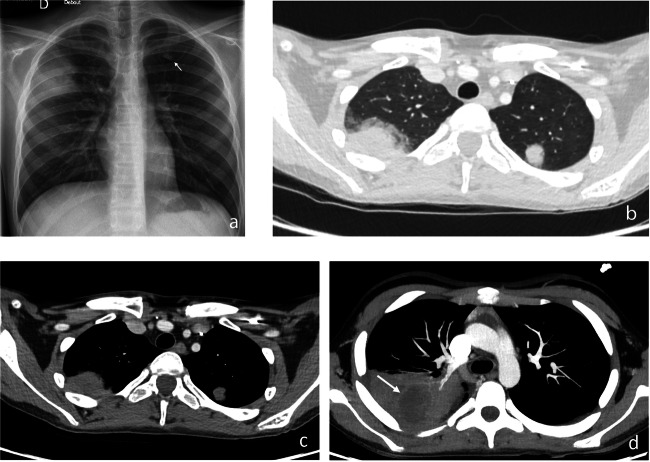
Proven invasive pulmonary aspergillosis in a 16-year-old boy with acute myeloblastic leukemia (AML) under chemotherapy. Chest X-ray (a) performed because of increasing thoracic pain after minor trauma evidences an extensive lung infiltrate with ill borders in the right upper lobe and a nodule below the chondro-costal junction of the 1st left rib (arrow). Axial chest CE-CT image in pulmonary (b) and mediastinal (c) window reveals that both nodules are partially surrounded by ground-glass opacity (“halo sign”). Diagnosis was confirmed by bronchoalveolar lavage. Maximum intensity projection (MIP) images of the follow-up chest CE-CT examination (d) in mediastinal window performed 4 days later show increasing extent of the right upper lobe mass, now with central necrosis
